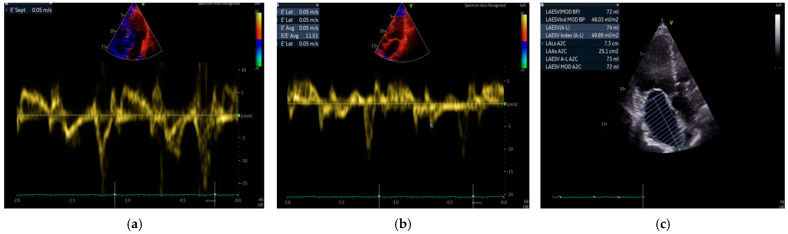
(a–c) Echocardiographic criteria for diastolic dysfunction diagnosis are based on three parameters: septal e’ (a), lateral e’ (b), and LAVI (c).
